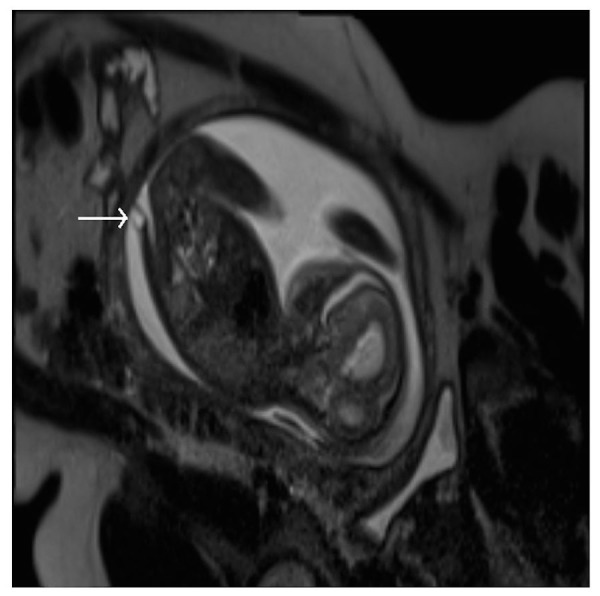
Neural tube defect Sagittal T2-weighted HASTE image demonstrating an open neural tube defect.
